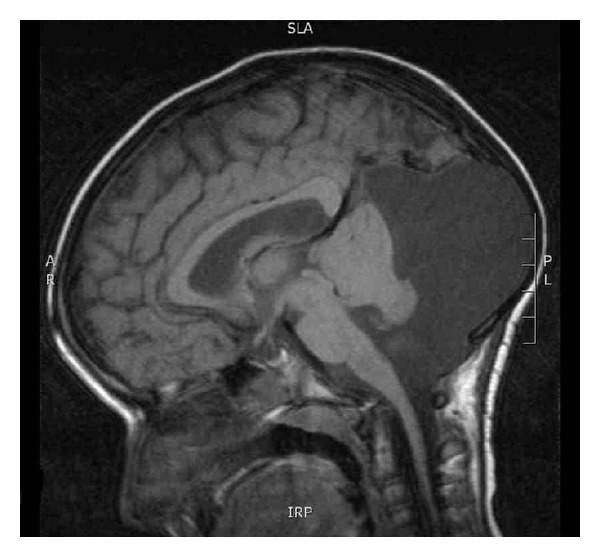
Sagittal MRI Head scans showing the presence of a large posterior fossa arachnoid cyst, this is predominantly left sided, causing mild midline shift and some distortion of the ventricular system. There is no hydrocephalus.
